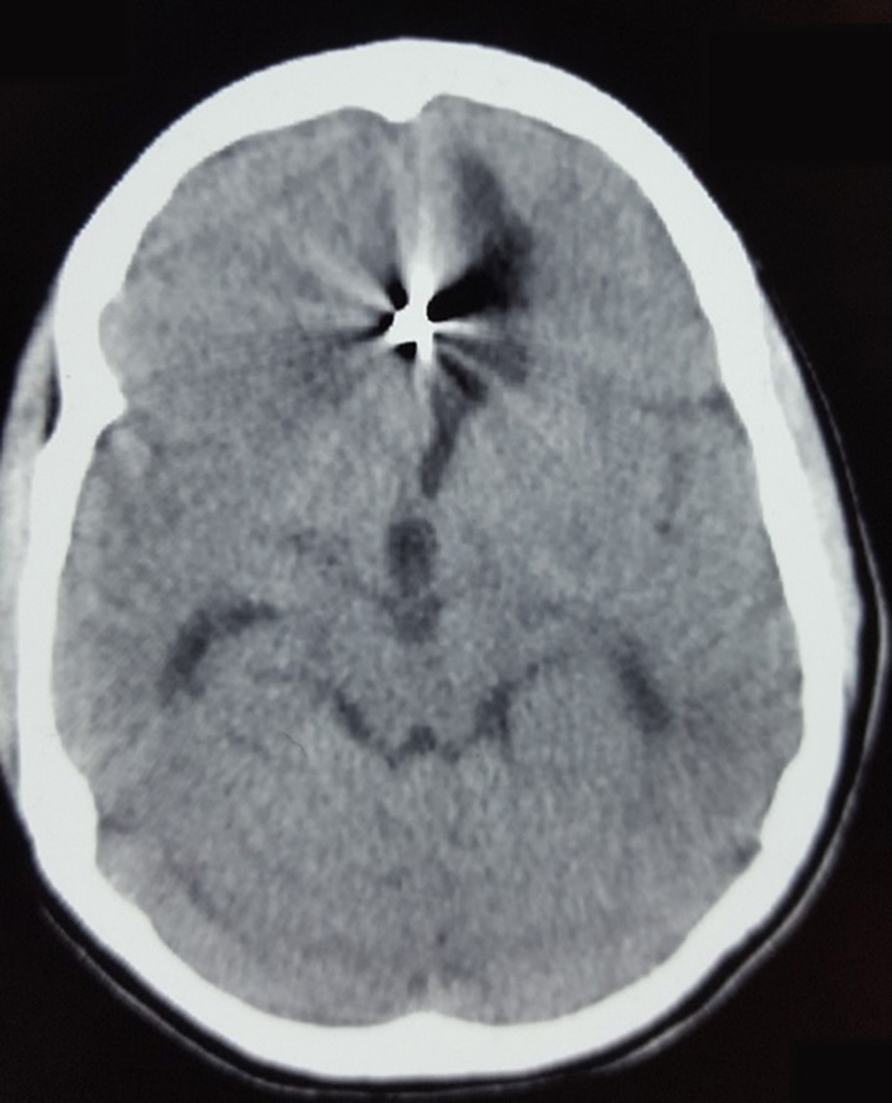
Axial CT scan showing metallic artefact due to coil along with chronic infarct frontal lobe.
